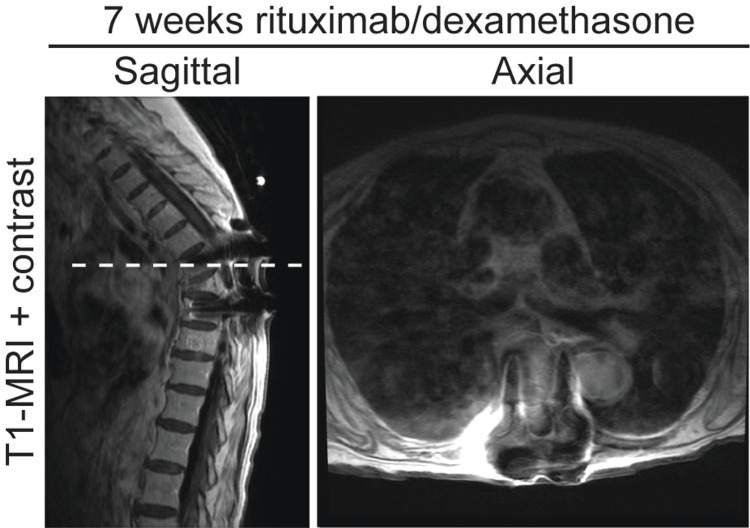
Tumor response to medical therapy with dexamethasone and rituximab. Contrast-enhanced T1-MRI sequences obtained seven weeks after initiating rituximab/dexamethasone medical therapy demonstrating tumor response to medical therapy. Dashed line represents level of axial cut. Study quality is limited by hardware artifacts.
